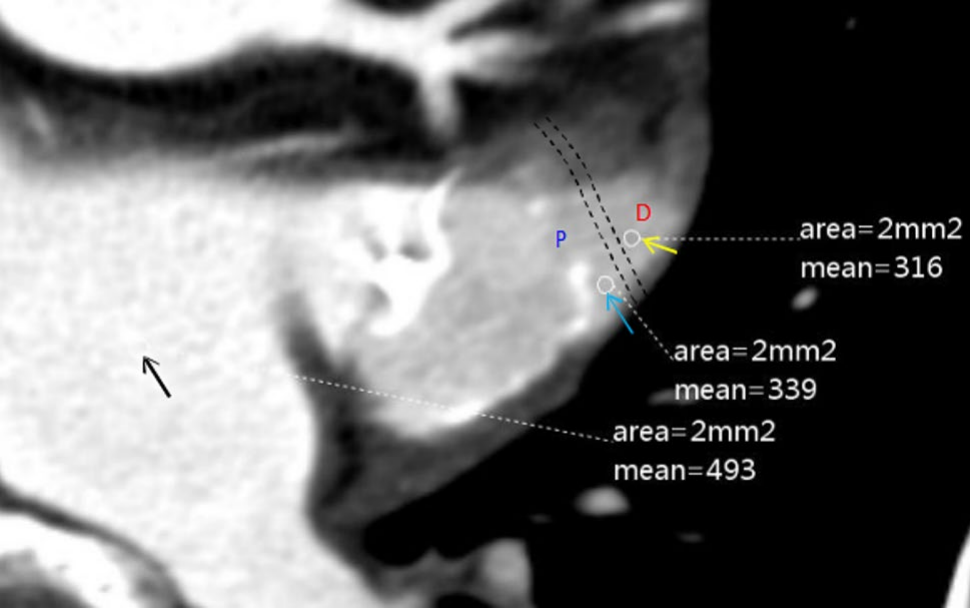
The measuring of Hounsfield units with the region of interest of 2 mm.^2^ on a contrast-enhanced CT image of contrast patency LAA: the distal area (D) of 316 HU (yellow arrow); the proximal area (P) of 339 HU (blue arrow); the center of the left atrium of 493 HU (black arrow)
